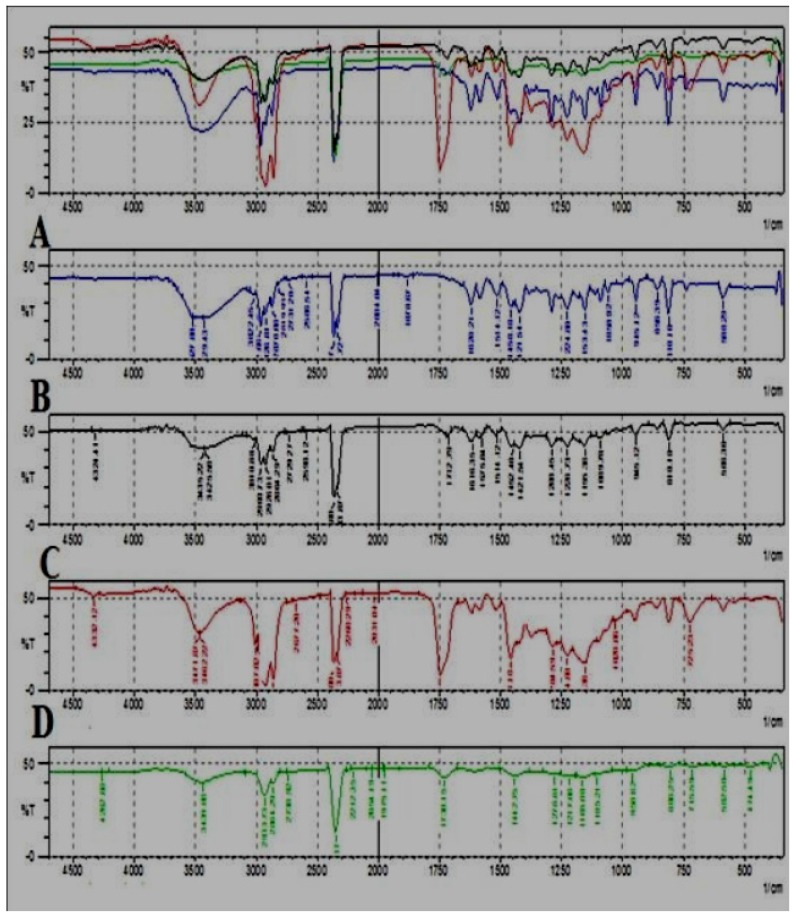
Comparative FTIR spectra of different oils of *T. ammi* L. extracted using different extraction techniques. (A) Hydrodistilled oil, (B) Solvent extracted oil, (C) SC-CO_2_ oil, (D) Ultrasonicated oil.
